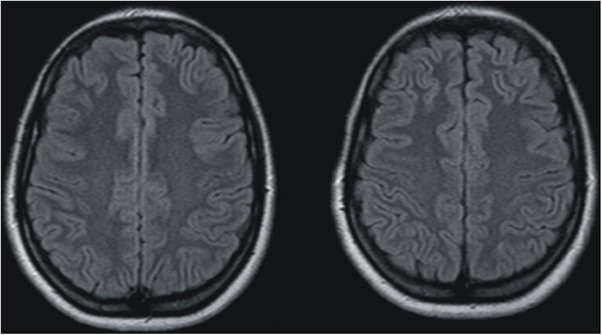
Follow‐up brain MRI showing the complete remission of signal abnormalities.
